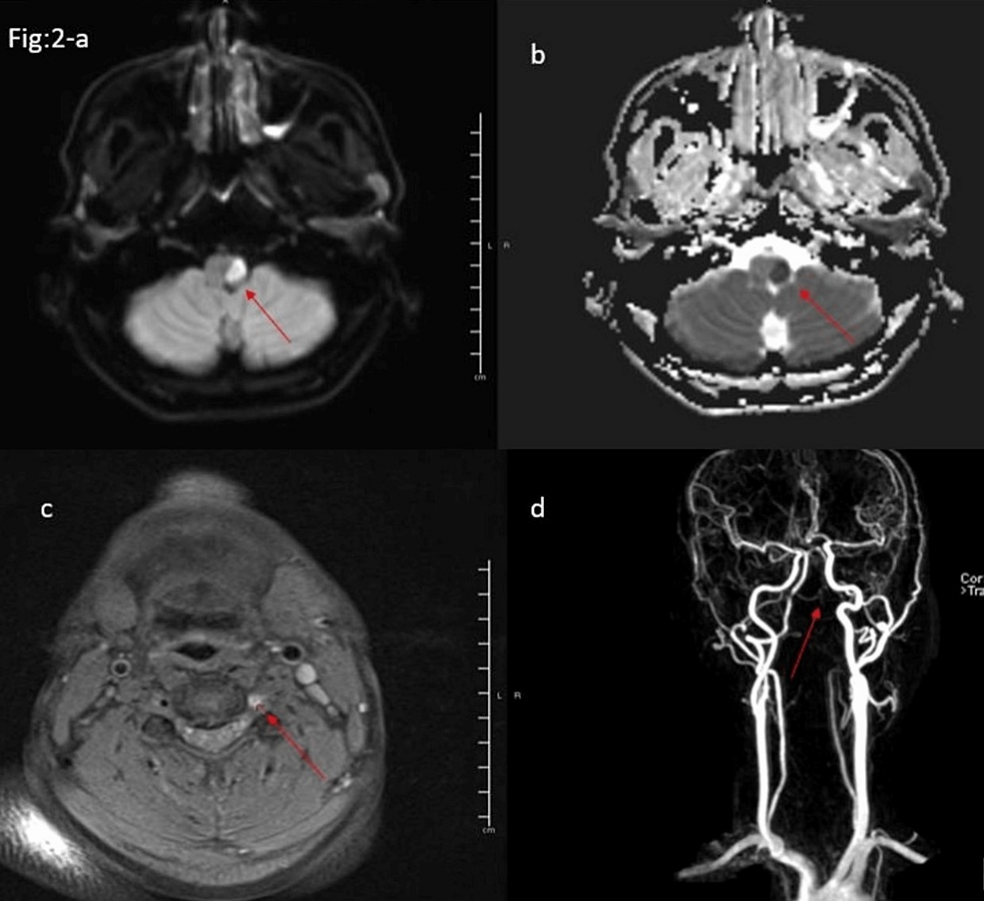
MRI of the head and MRA of the head and neck vessels, showing a left lateral medullary infarction and a dissection of the left vertebral artery. MRI of the head (a and b) shows an acute infarct in the left medulla. The MRI Axial T1 fat saturation image (c) suggests a circumferential dissection of the left vertebral artery, and MRA of the neck (d) identifies no significant flow within the left V3 and V4 segments.
